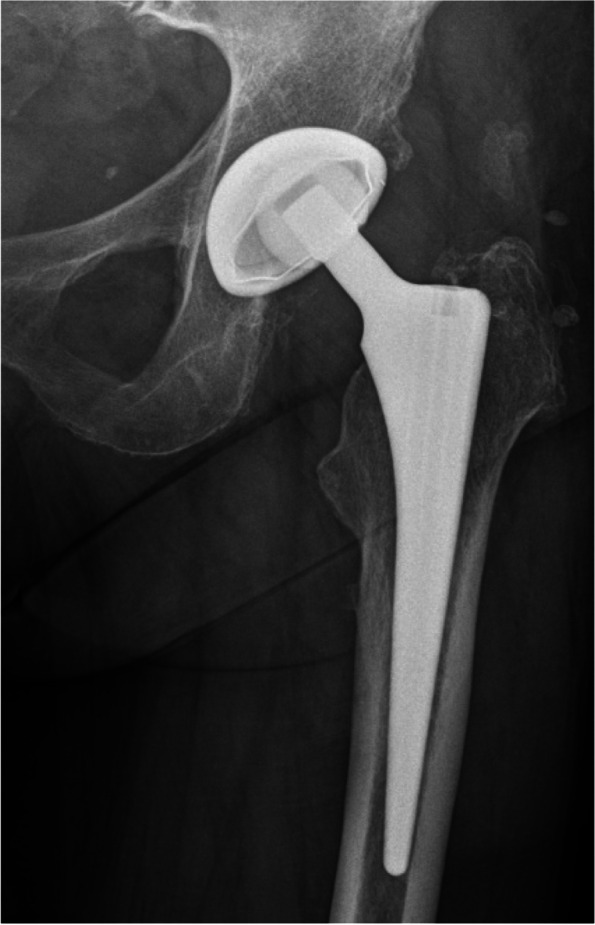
Plain radiograph shows asymmetric polyethylene wear of the inlay
